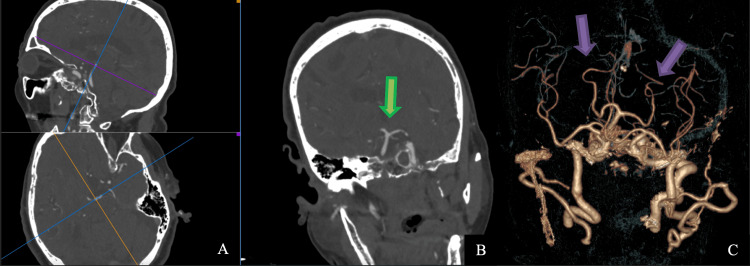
Postoperative CT-angiography images A, B: Multiplanar reconstruction (MPR) images with complete recanalization of the tip of the basilar artery, marked with a green arrow in B. C: The 3D reconstruction of the CT angiography shows perfect filling with a contrast of both posterior cerebral arteries (PCAs), marked with purple arrows.
